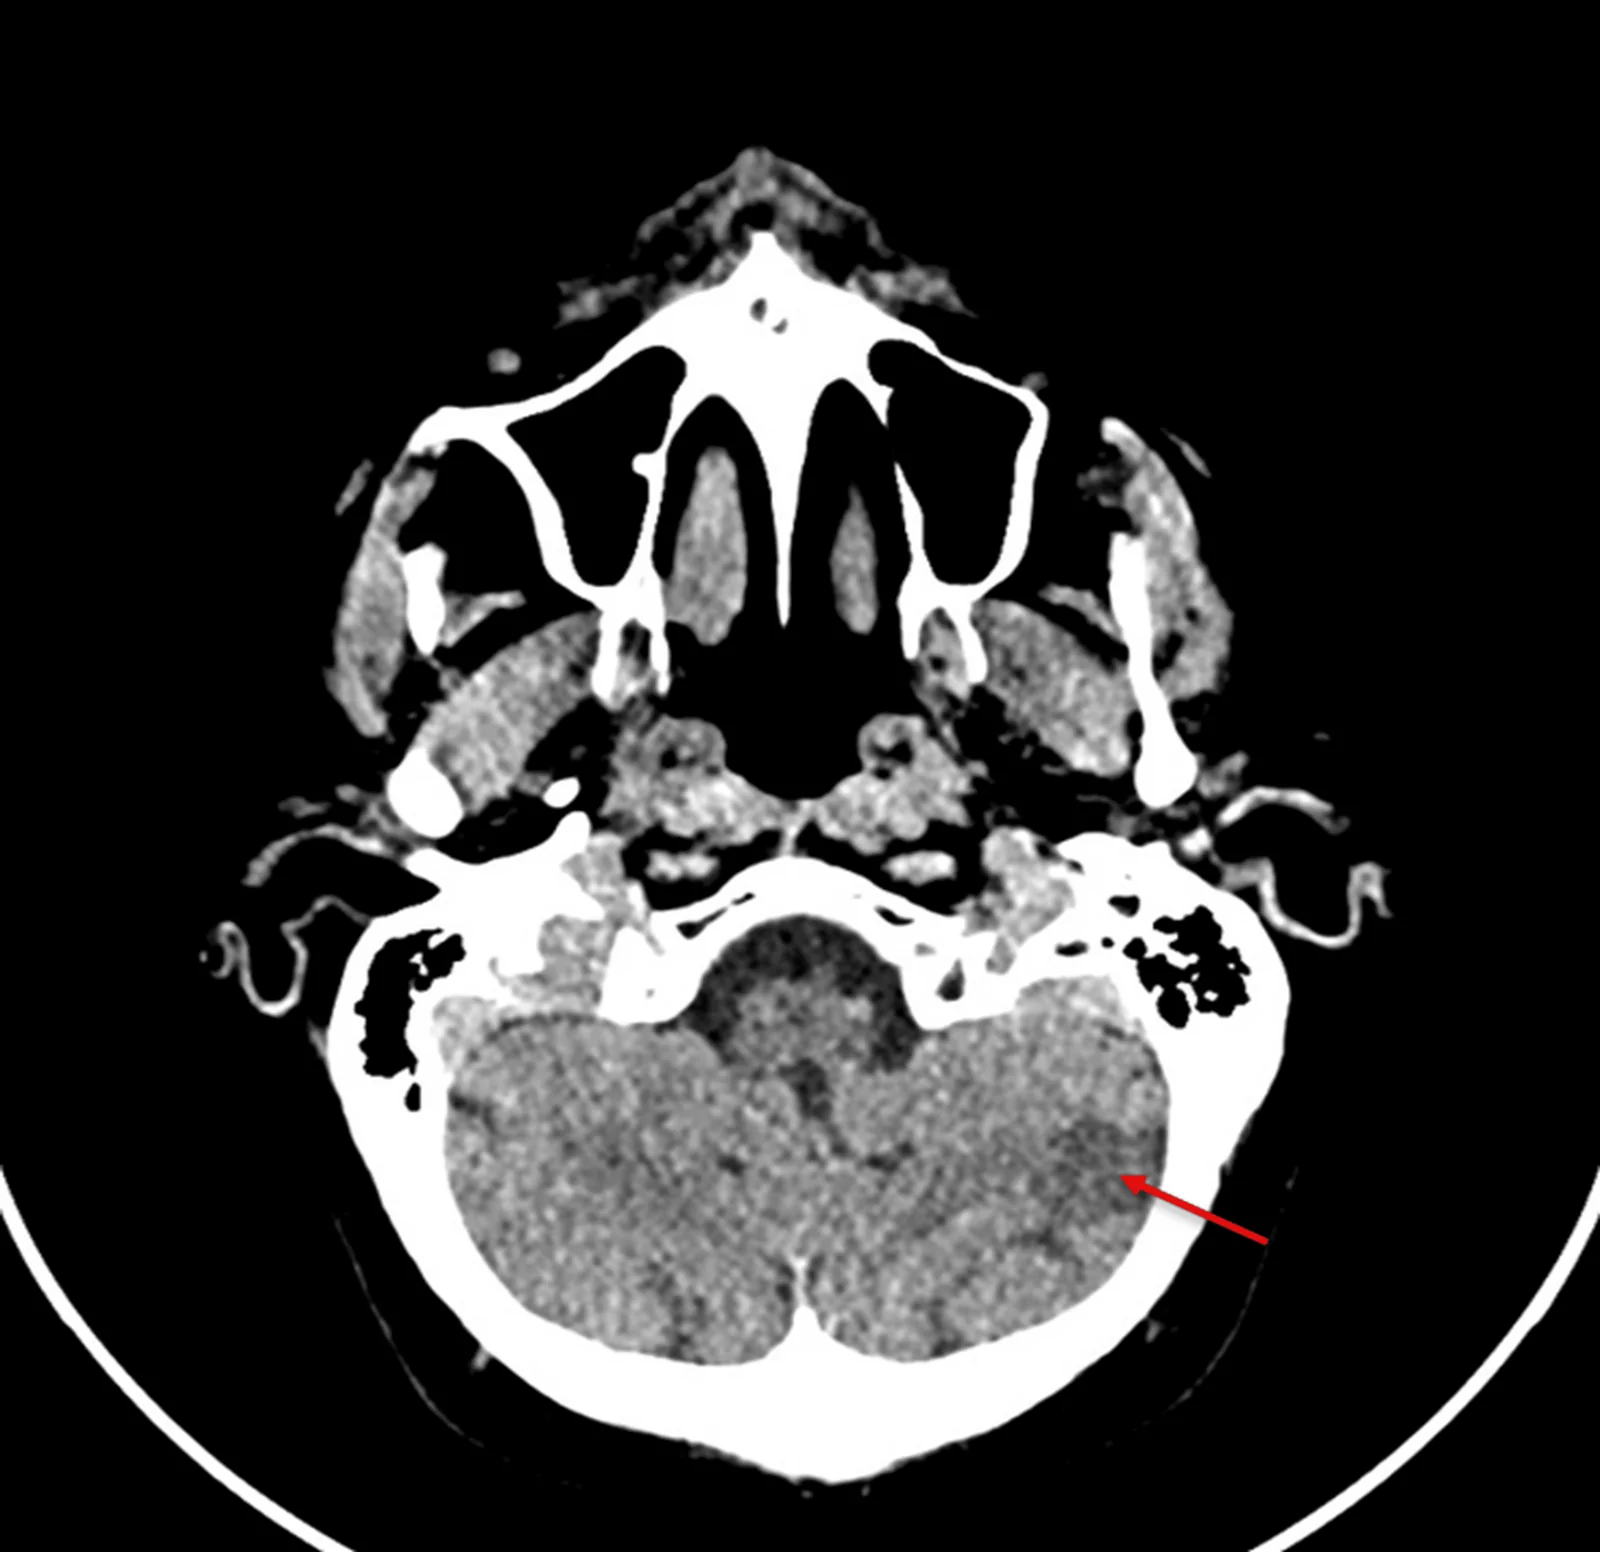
Non-contrast head computed tomography showing a left cerebellar hypodensity (red arrow)
